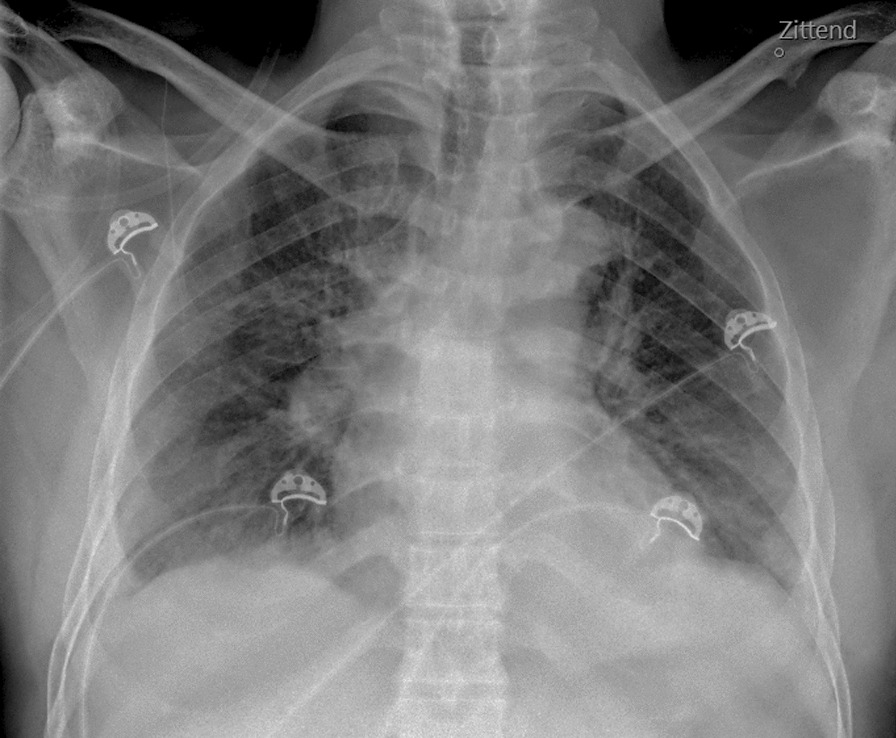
Chest X-ray with pleural effusion and bilateral peripheral consolidations
